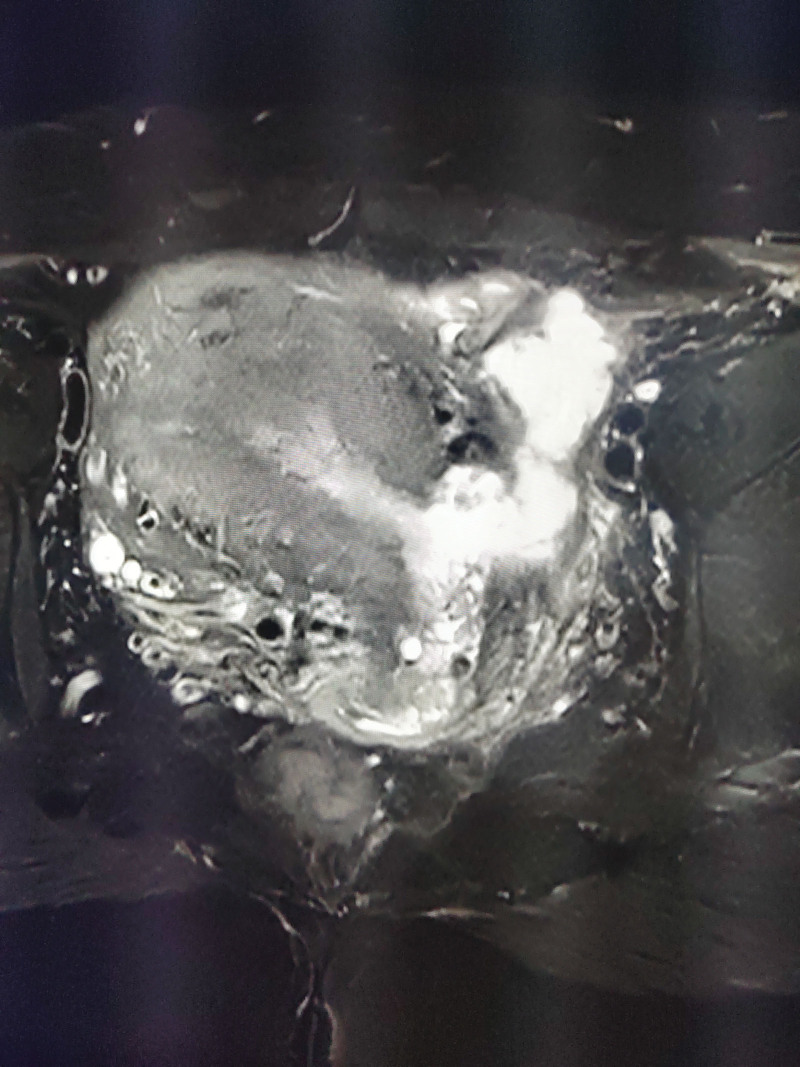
On MRI, the lesion appeared as a localized mass and was also found to be linked to the uterine cavity, with evidence of myometrial discontinuity. MRI = magnetic resonance imaging.
